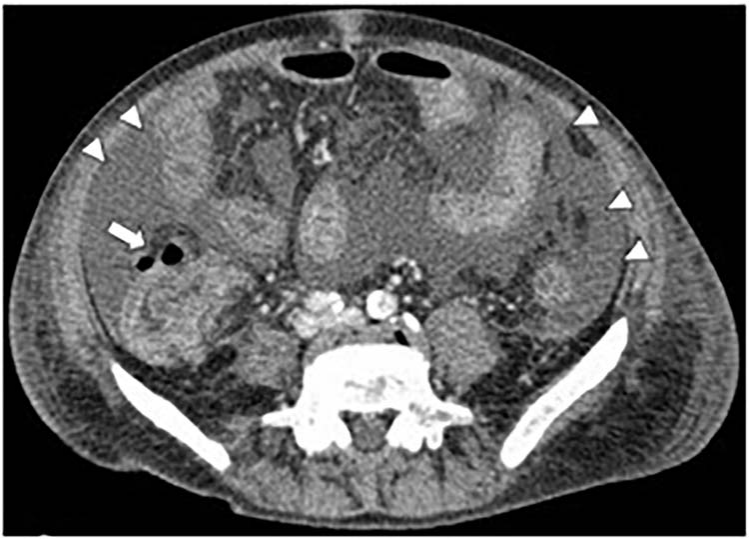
CT image of ascites (arrowheads) and free air (arrow).
